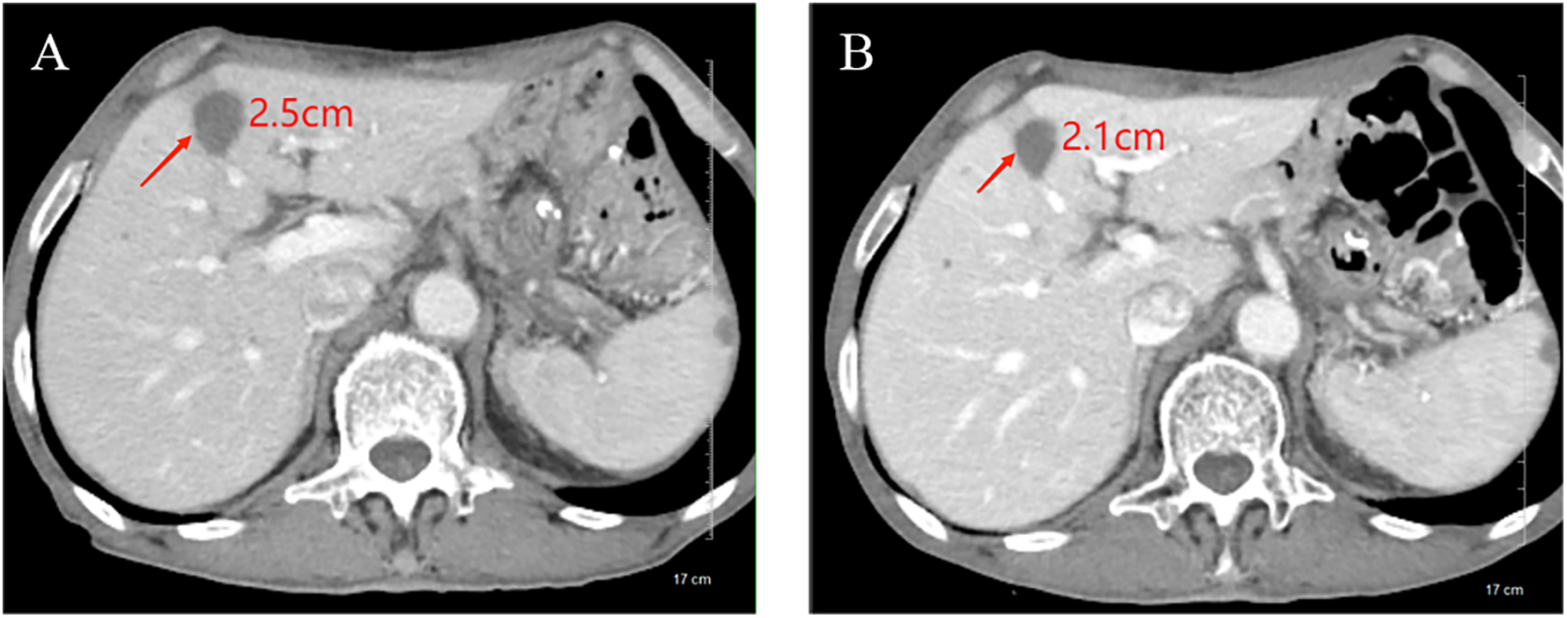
Comparison of longest diameter of liver metastases on CT images before and after chemotherapy plus ICI therapy. (A) Before treatment, the longest diameter of liver metastases was about 2.5cm; (B) After treatment, the longest diameter of liver metastases was reduced to 2.1cm.
